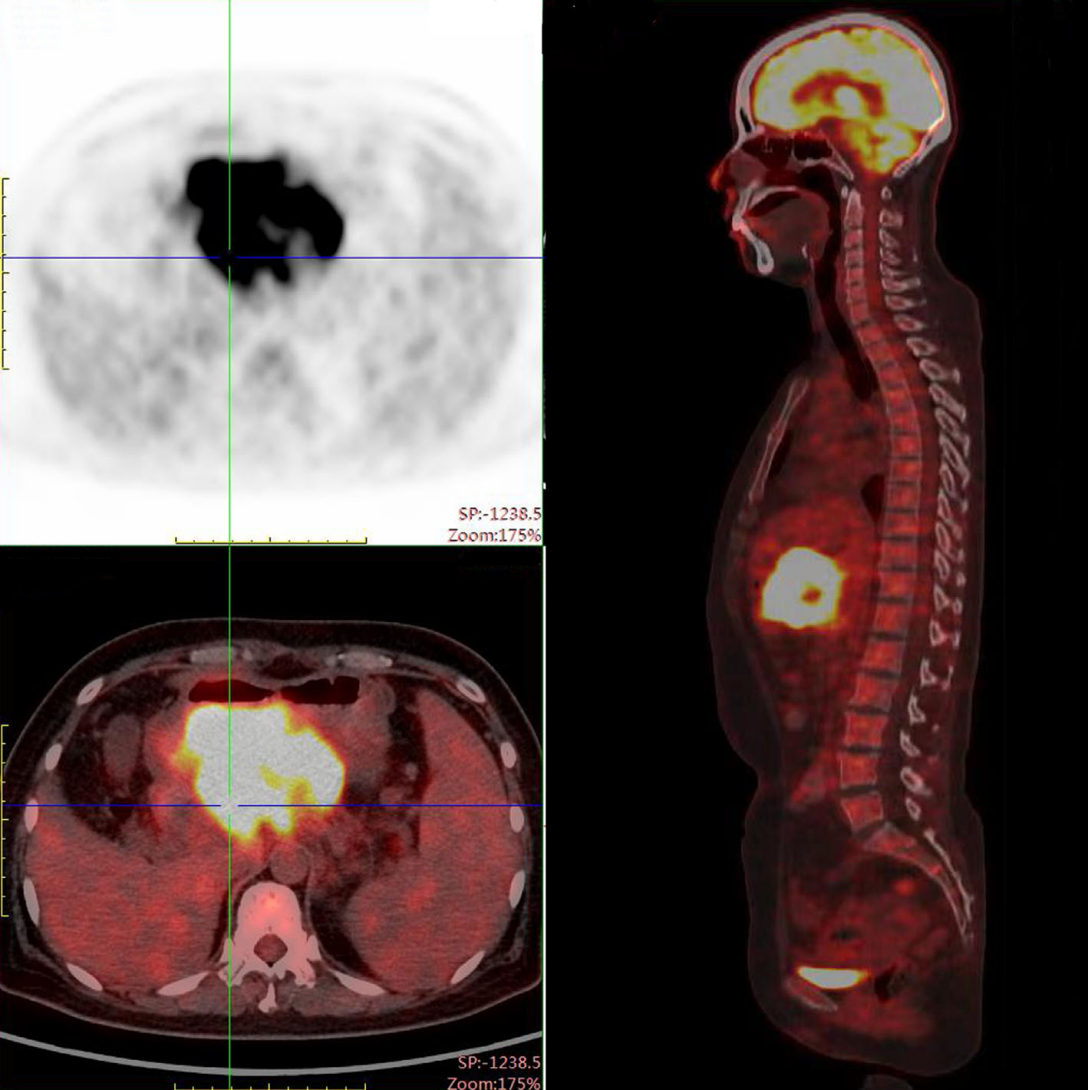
PET-CT scan demonstrated a mass measuring 105 x100 x 70 mm with peripheral elevated FDG avidity in the neck of the pancreas.
